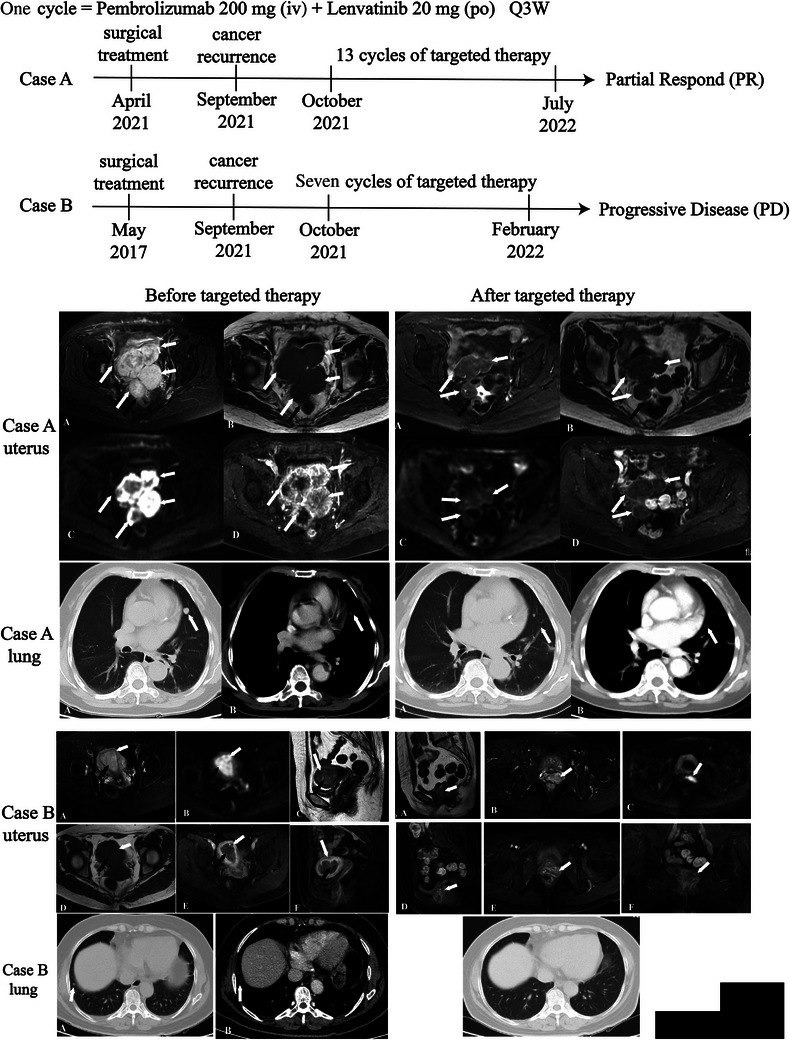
Patient with treatment plan and computed tomography (CT), magnetic resonance imaging (MRI) changes before and after treatment used in the study.
